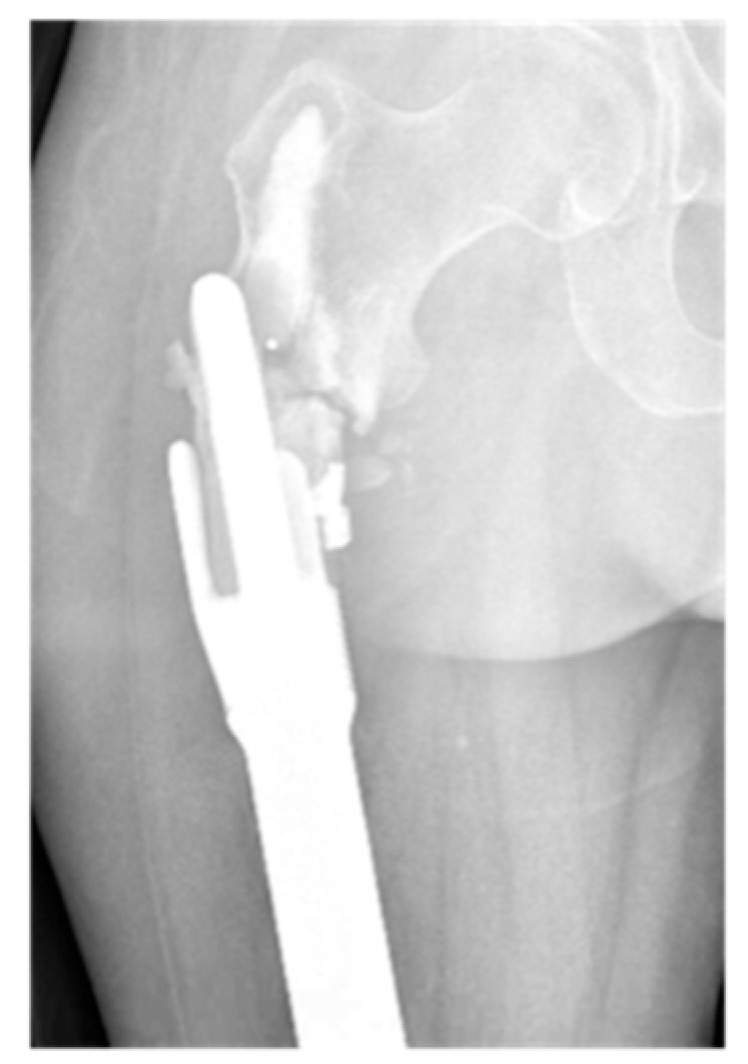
AP radiograph showing grade 6 loosening of EPR with periprosthetic fracture.
